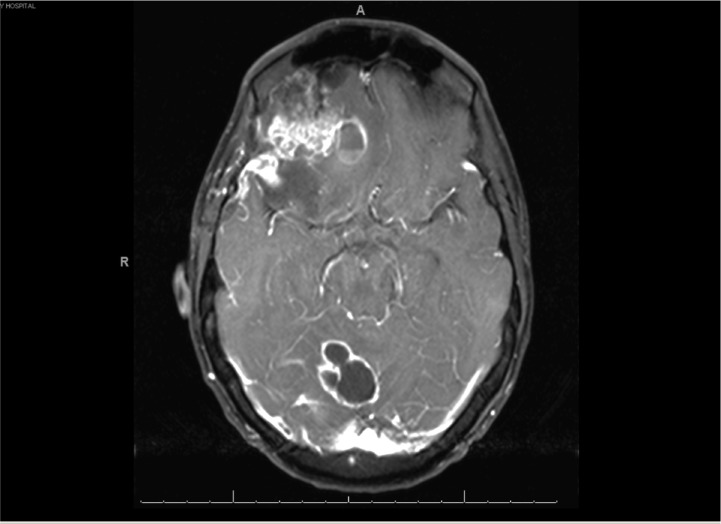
MRI Five Months After Initial Presentation Marked progression in a multiple ring-enhancing lesions with vasogenic edema.
